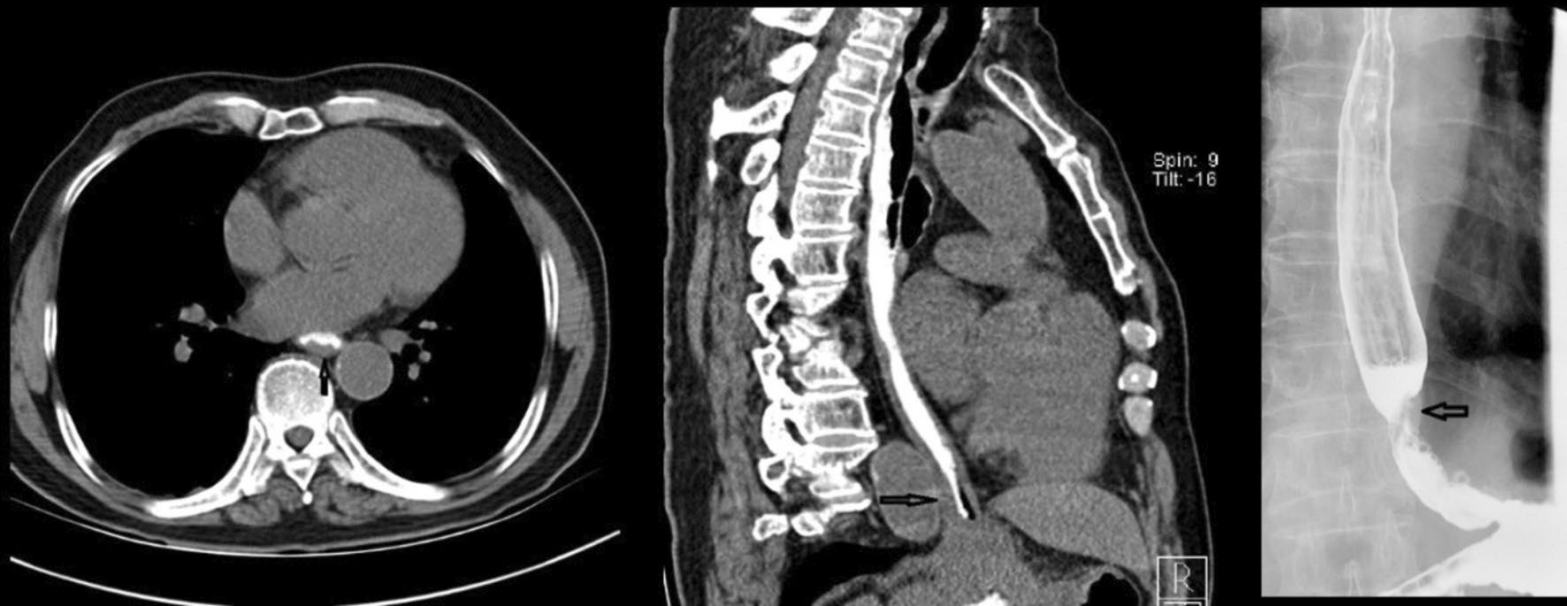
Computed tomography (CT) image of the esophagus filled with lotus root powder. A Thickening of the wall of the lower segment of the esophagus, especially the thickening of the posterior wall. B CT sagittal image of the esophagus filled with lotus root powder, showing thickening of the lower esophagus wall and the back wall. C Esophageal barium meal contrast image, showing lumen stenosis in the lower segment of the esophagus, which was consistent with the CT images
